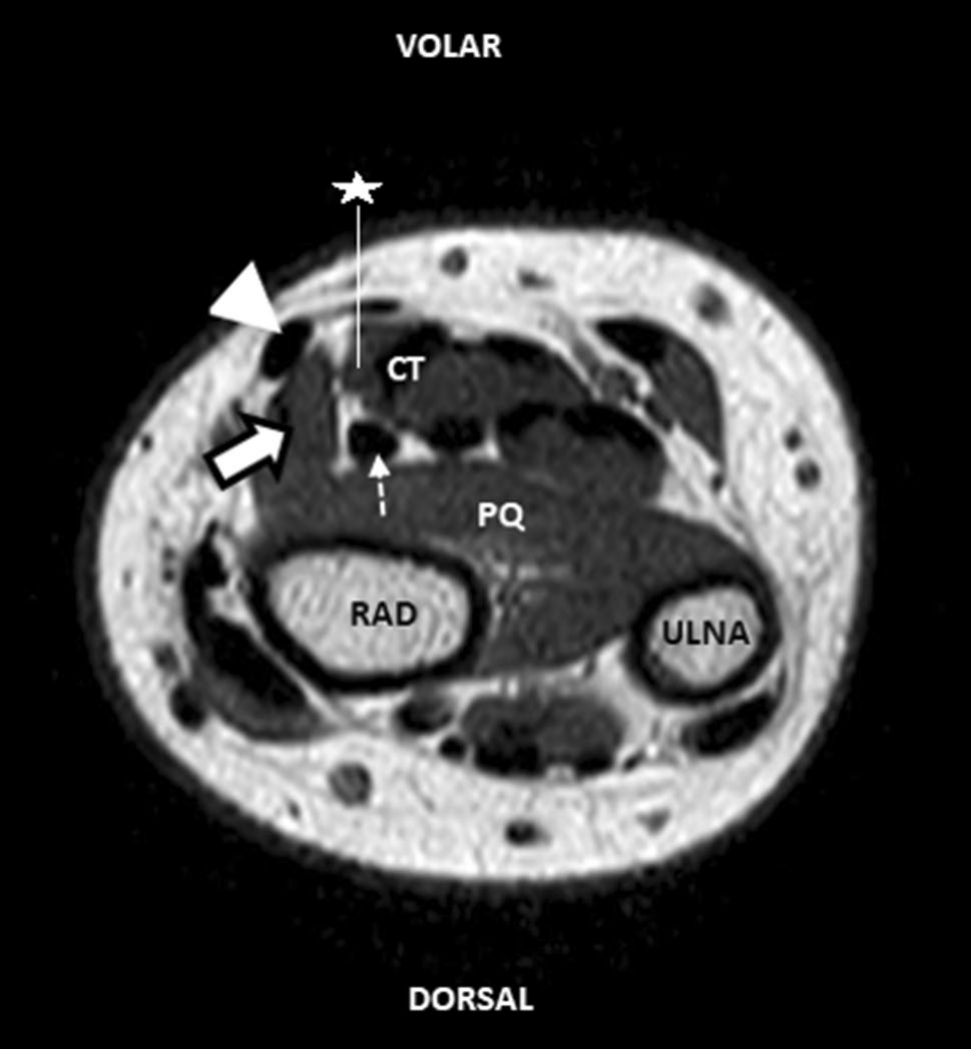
Axial T1W TSE MR image of the wrist; m. flexor carpi radialis tendon (arrowhead), m. flexor carpi radialis brevis (arrow), m. flexor pollicis longus (dotted arrow), carpal tunnel contents (CT), m. pronator quadratus (PQ), radius (RAD), n. medianus (star).
